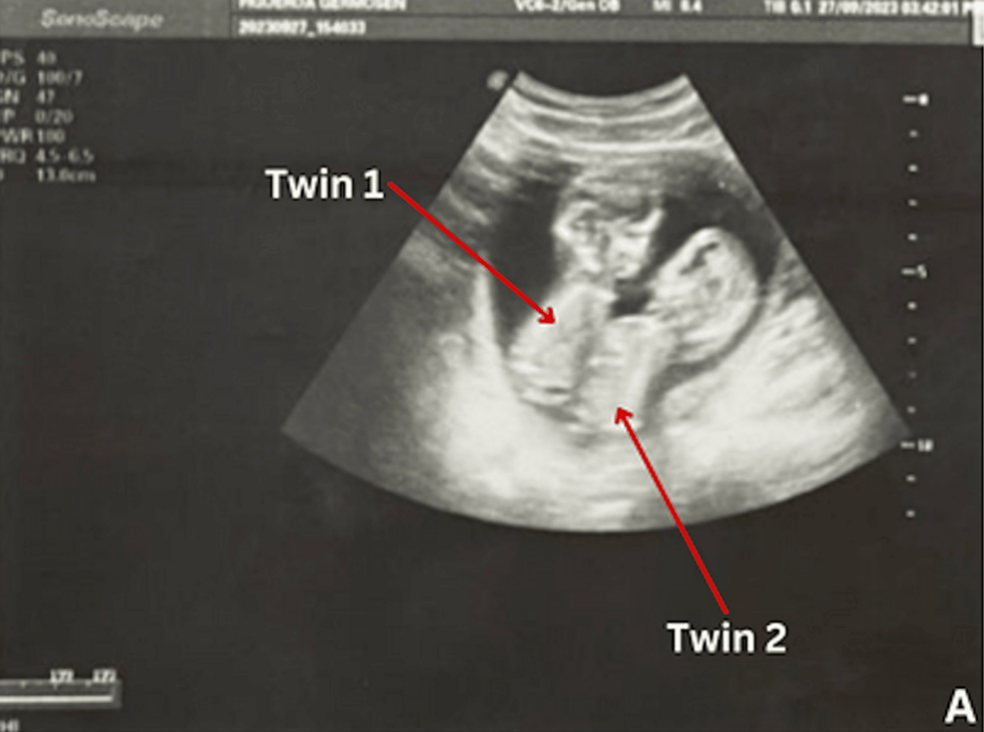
Prenatal sonography image showing the union between both fetuses at thoracic level and upper abdomen, sharing a single heart, showing total separation of heads (located face to face), pelvis and upper and lower limbs. The patient was diagnosed at this point with a twin gestation of thoraco-omphalopagus conjoined twins at 14.2 weeks.
